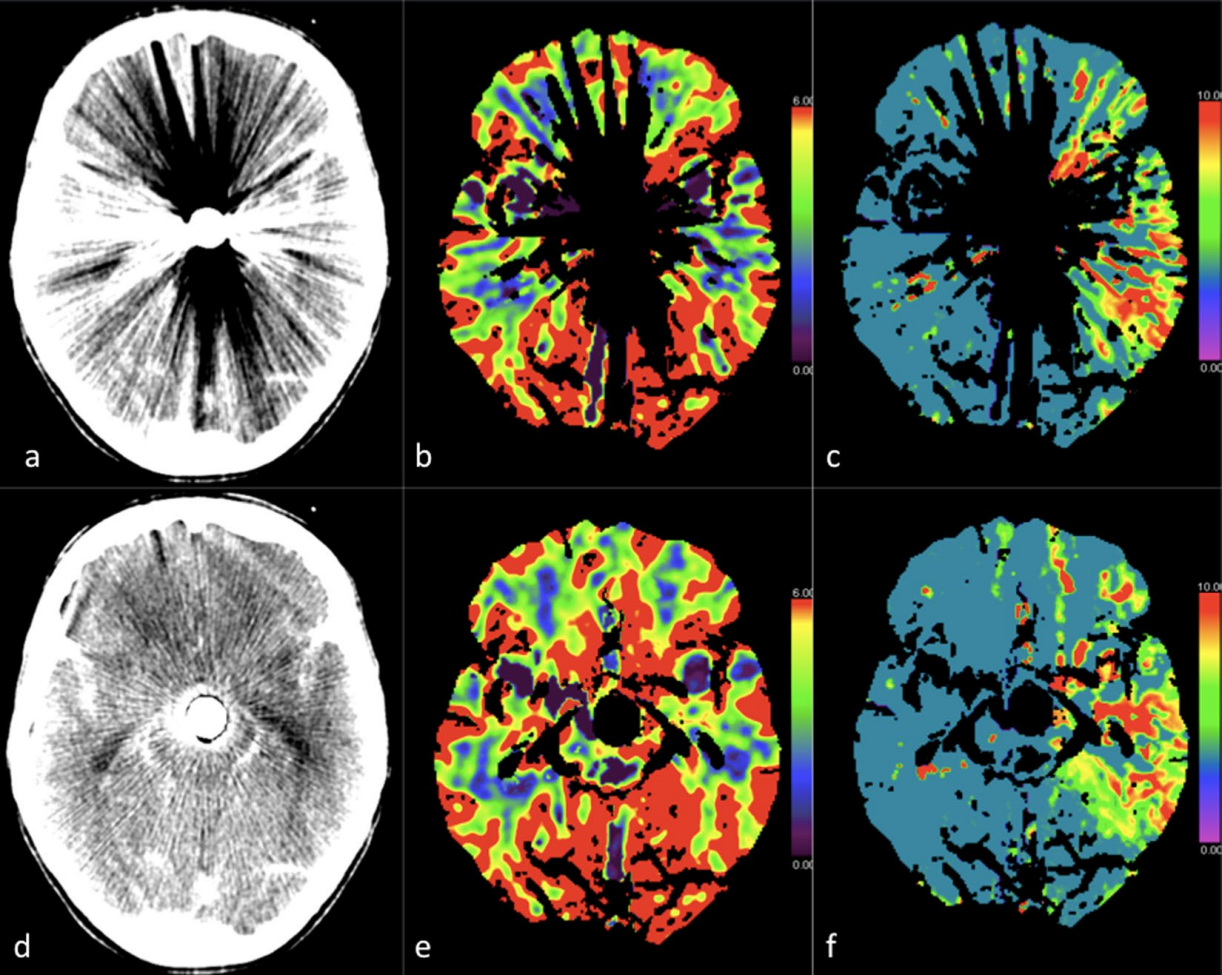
CT perfusion study of a 60-year-old female patient after coiling of a ruptured basilar tip aneurysm. The upper panel (a–c) show images before, and the lower panel (d–f) show images after iMAR application. Obvious metal artifact reduction was apparent after iMAR application, which improved the detection of MTT prolongation in the left temporal lobe (c,f) and increased rater confidence. Rater confidence in analysing the CBV (b,e), which showed no asymmetry even after artifact reduction, was also increased. Note: A hypodense ring artifact surrounded by a hyperdense ring was observed around the metal in the source images processed with iMAR (d).
